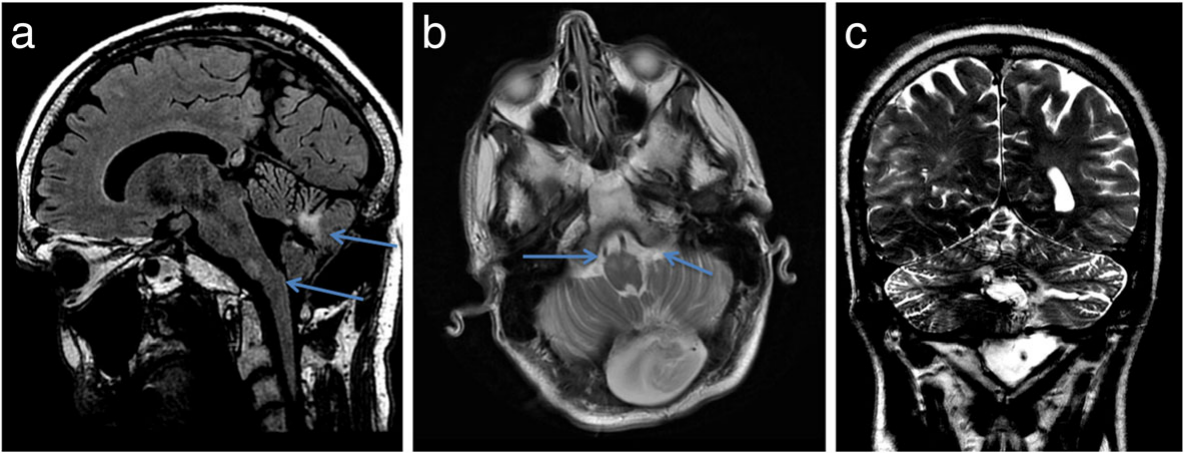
Sagittal FLAIR (a) showing (blue arrows) high signal affecting inferior olivary nuclei, and gliosis and malacia at the surgical bed with presumed damage to central tegmental tract and dentate nuclei. Axial T2-weighted (b) showing high signal medulla bilaterally, at the level of the inferior olivary nuclei. Post-operative pseudomeningocoele is also evident. Coronal T2-weighted (c) further highlights post-operative damage to cerebellum involving central tegmental tract and dentate nuclei with gliosis and hemosiderin deposition
